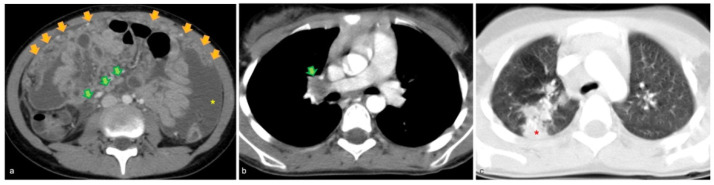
Axial images of the contrast enhanced whole-body computed tomography scan of the same child confirming the omental cake and ascites (orange arrows and yellow asterisk in a), enlarged abdominal and mediastinal lymph nodes (green arrows in a,b) with a necrotic core in this latter area, and a pulmonary consolidation in the right upper lobe (red asterisk in c).
